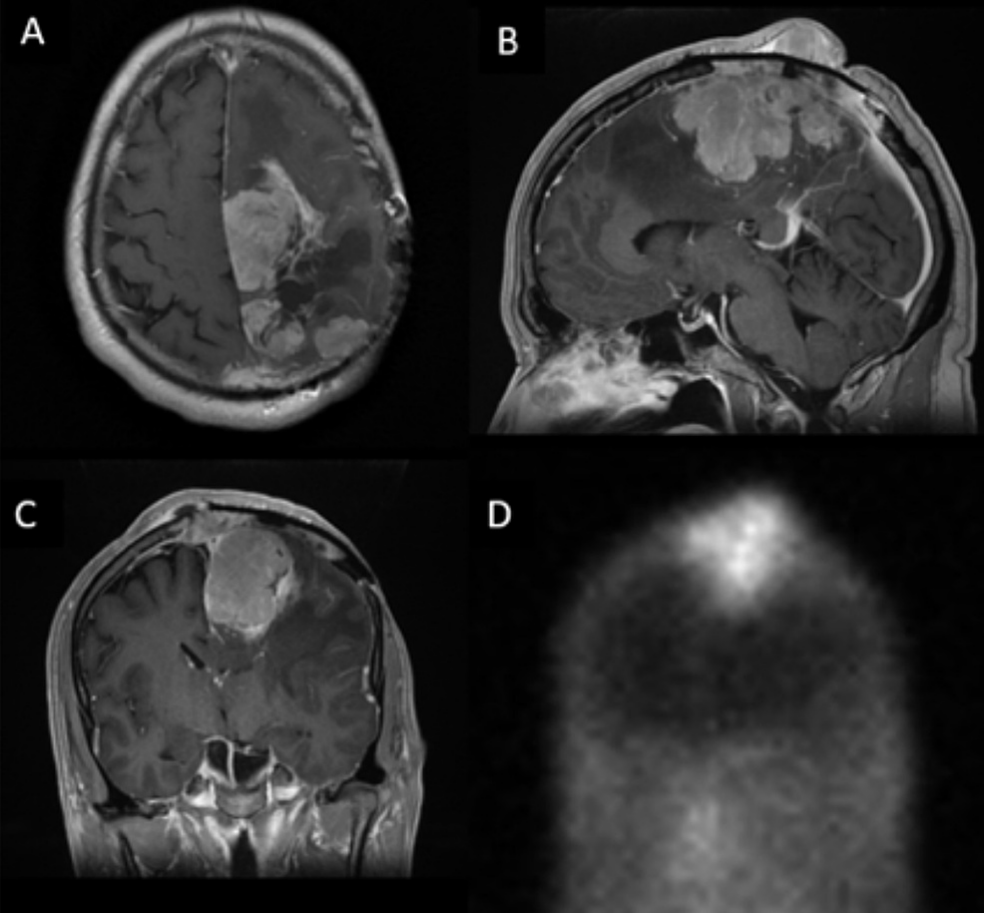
Magnetic resonance imaging T1-weighted contrast-enhanced MRI images in axial, sagittal, and coronal planes demonstrate a large left parietal meningioma with significant mass effect and invasion of the superior sagittal sinus and skull (A, B, C).  Nuclear medicine study demonstrates radiolabeled octreotide uptake within tumor on the coronal view (D).
